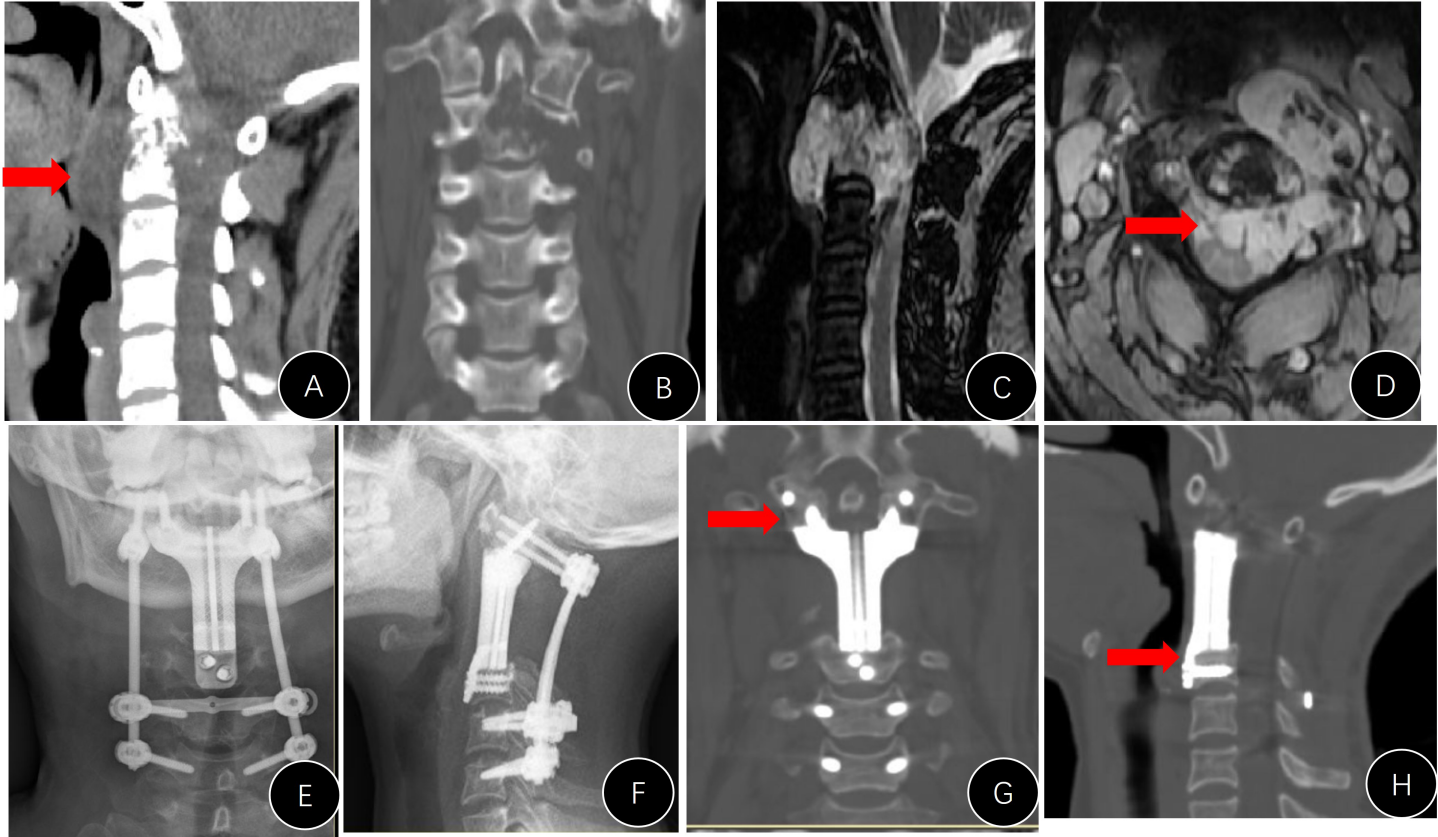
Presentation of case #B15. (A–D) Preoperative CT and MRI images; (E, F) postoperative x-rays; (G, H) CT reconstruction films 1 year later, indicating that the implant was well attached to the C1 lateral mass and superior endplate of C3.
